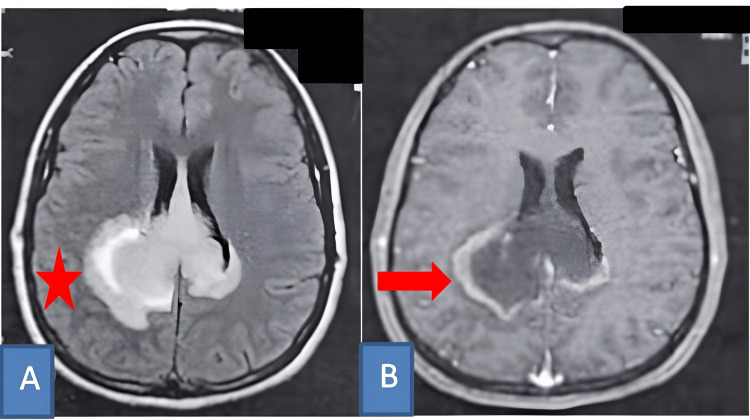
Magnetic resonance imaging (MRI) of the brain showing a neoplastic lesion centered in the splenium of the corpus callosum with parietal lobe extension. (A) Axial FLAIR sequence demonstrating a hyperintense lesion (red star). (B) Axial T1-weighted sequence after gadolinium administration showing a hypointense lesion with peripheral contrast enhancement (red arrow). The imaging features are consistent with a low-grade glioma with partial enhancement and extension toward the parietal white matter. FLAIR: fluid-attenuated inversion recovery.
